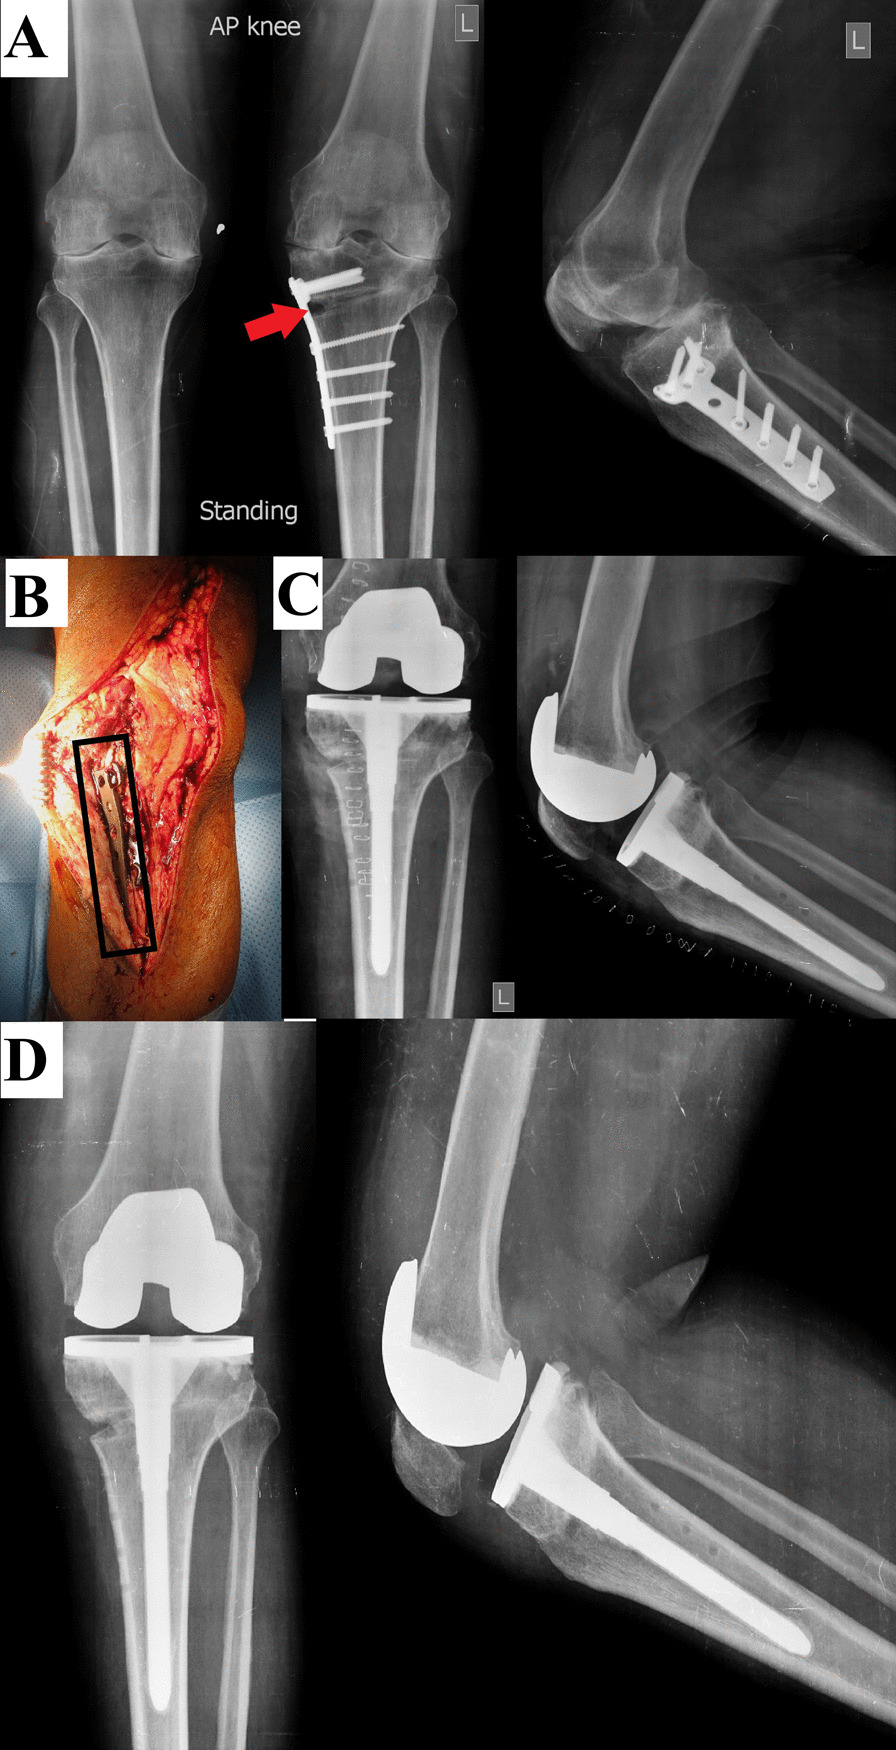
Male patient, 59 years old. A MWOHTO performed in 2014 using a locked T-plate, preoperative radiographs showed signs of nonunion (red arrowhead). B Intraoperative images while the knee was approached through a medial parapatellar approach, showing the extent of the medial plate. C Immediate postoperative after conversion to TKA using a PS implant and a tibial stem in February 2017. D After three years of follow-up, although the medial HTO gap did not fully unite, the implants showed preserved proper positioning
